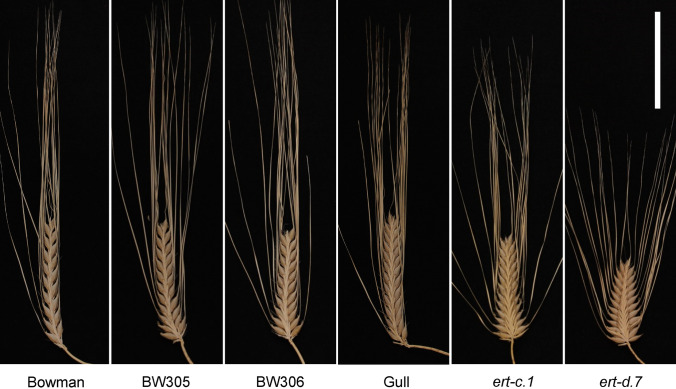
Spike phenotypes of Bowman, near-isogenic lines BW305 and BW306, Gull and original mutants *ert-c.1* and *ert-d.7*. The two *ert* mutants were induced by X-rays in the cultivar Gull in 1937 and 1941, respectively (www.nordgen.org/bgs). The near-isogenic lines BW305 and BW306, were generated from recurrent backcrosses between cultivar Bowman and *ert-c.1* and *ert-d.7*, respectively (Druka et al. 2011). Scale bar: 5 cm
